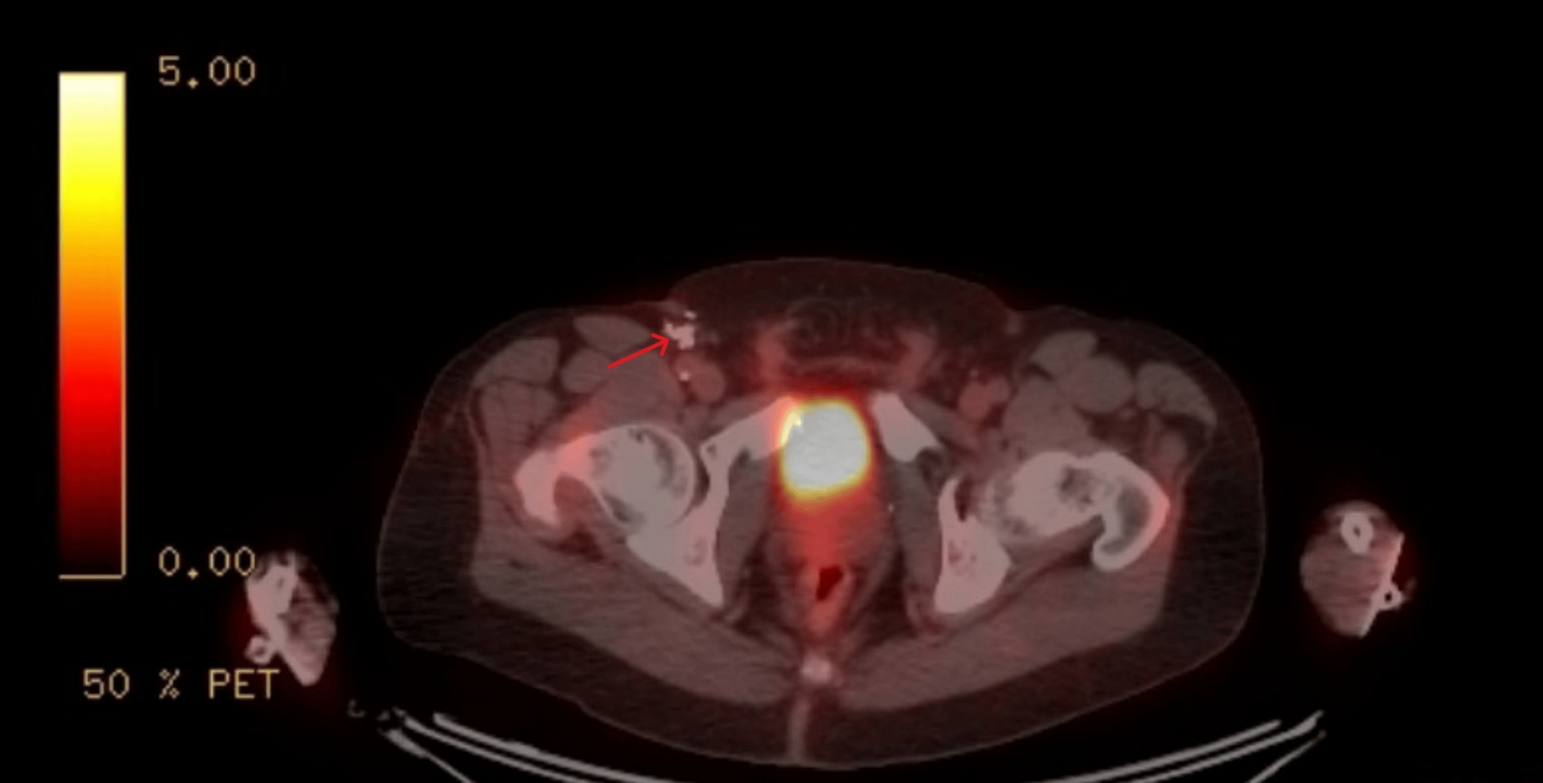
PET-CT after antibiotic therapy showing resolution of right inguinal lymphadenopathy, with post-surgical changes
